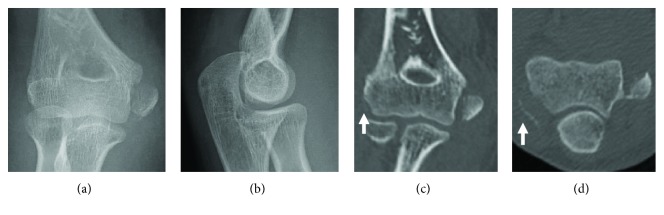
Preoperative X-ray (a, b) and CT (c, d) of the right elbow. (a) AP view. (b) Lateral view. (c) Coronal plane. (d) Axial plane. Thin bony fragment of the lateral epicondyle (arrow) was observed in (c) and (d).
